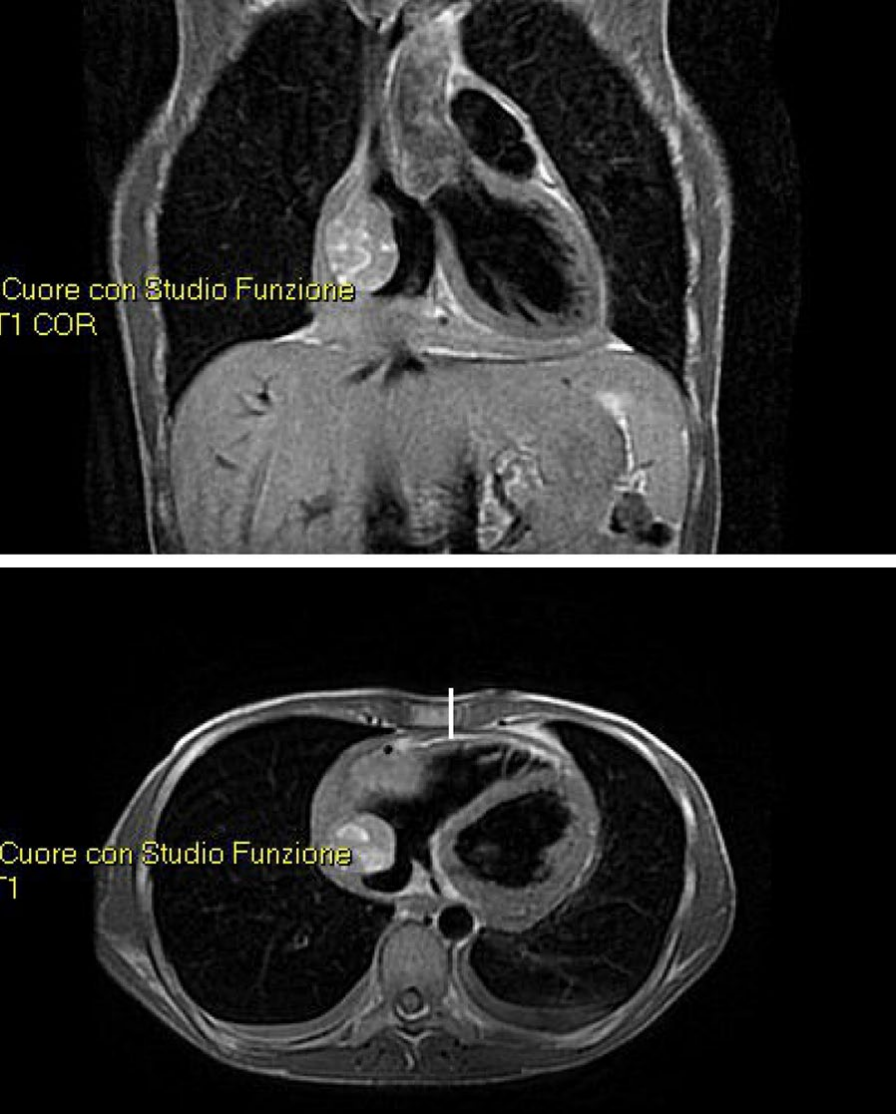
Nuclear magnetic resonance imaging before neoadjuvant therapy with docetaxel.
